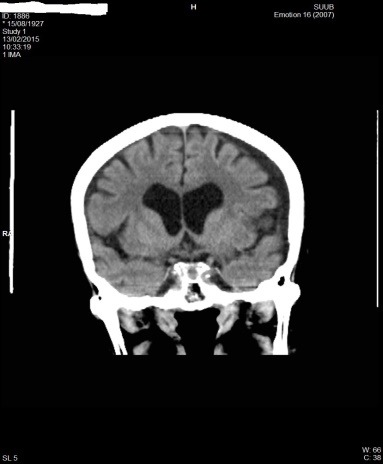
CT aspect (coronal plane) with left F-T hygroma in patient P.M. of 87 years old
